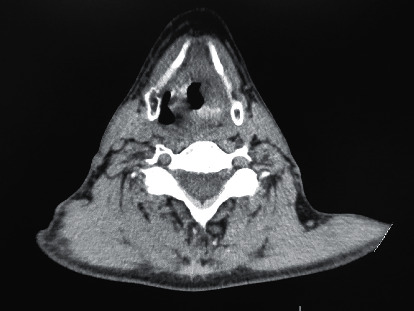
CT scan (axial view): an infiltrative mass at the left supraglottic area with extension to the left TVC.
